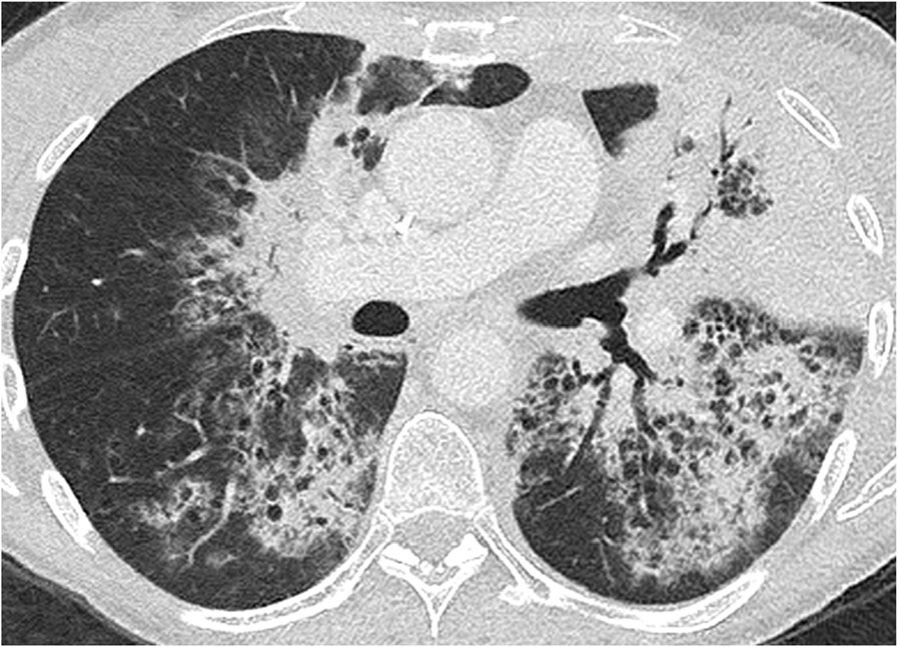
Immune-related pneumonitis presenting as an organising pneumonia pattern in a patient with metastatic lung cancer that occurred after 13 cycles of anti-PD1 therapy. This axial CT image in lung windowing shows multifocal alveolar consolidations in a subpleural and peribronchovascular location, predominating at the level of the left upper lobe. Although suggestive of a diagnosis of organising pneumonia, infectious or tumoural lesions were excluded by means of a brochoalveolar lavage. Note that numerous round lucencies are visible within the alveolar consolidations, corresponding to associated centrilobular emphysema in this heavy smoker patient
